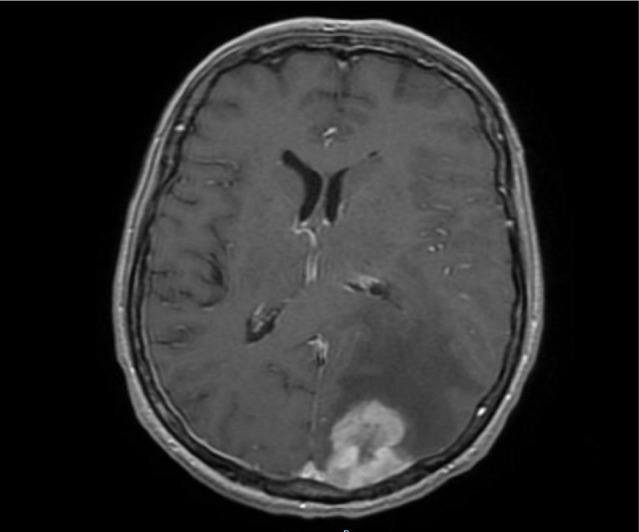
Gadolinium-enhanced T1-weighted MRI scan, axial section, showing a large left parieto-occipital extra-axial tumor, with a particular dural tail sign and peritumoral edema compressing the occipital horn of the left lateral ventricle and causing midline shift, suggestive of meningioma.
